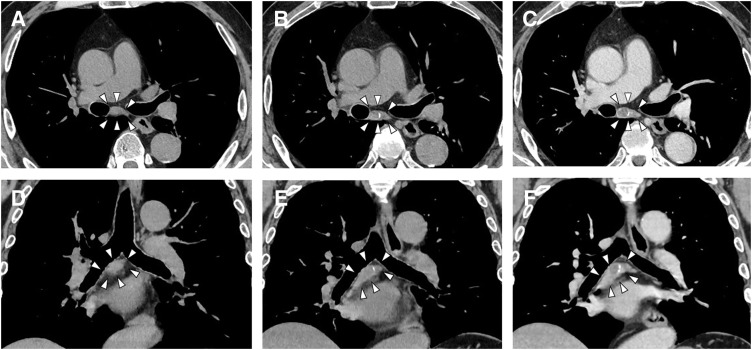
A mass (arrowheads) in the subcarinal region of the mediastinum. (A, D, non-contrast) CT images obtained at the time of surgery for thyroid cancer. (B, C, non-contrast) and (E, F, contrast-enhanced) CT images obtained 2 years after surgery.
